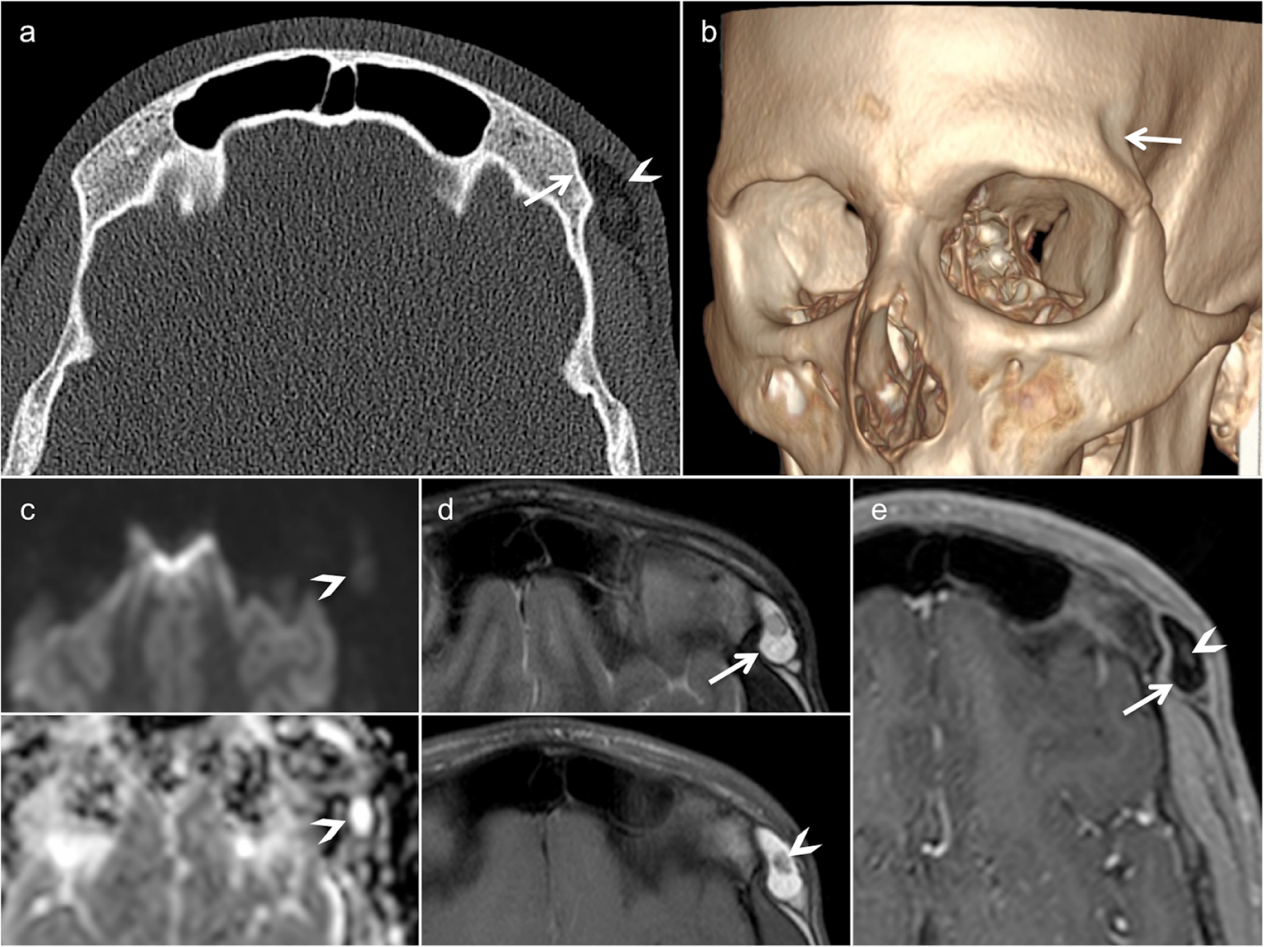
DC with bone remodelling in a 14-year-old male with lateral supraorbital soft tissue swelling and induration. a Axial CT (bone window) shows well-demarcated cystic subcutaneous lesion (*arrowhead*) with frontal bone scalloping and no cortical erosion (*arrow*). b Three-dimensional CT VR reconstruction shows bone remodelling in the supraorbital left area (*arrow*). c DWI reveals high signal on the b = 1000 image (*arrowhead*, *upper image part*) and high signal on the ADC map (*arrowhead*, *lower image part*, ADC = 2.5 × 10^−3^ mm^2^/s), compatible with no restricted diffusion and characteristic of a DC. d Peripheral lipid density on T2 (*arrow*, *upper image part*) and T1 with low signal (*arrow* in e) in gadolinium-enhanced coronal fat-saturated T1. Central soft tissue density on T1 (*arrowhead* in *lower image part*) with no contrast enhancement (*arrowhead* in e), corresponding to squamous debris
